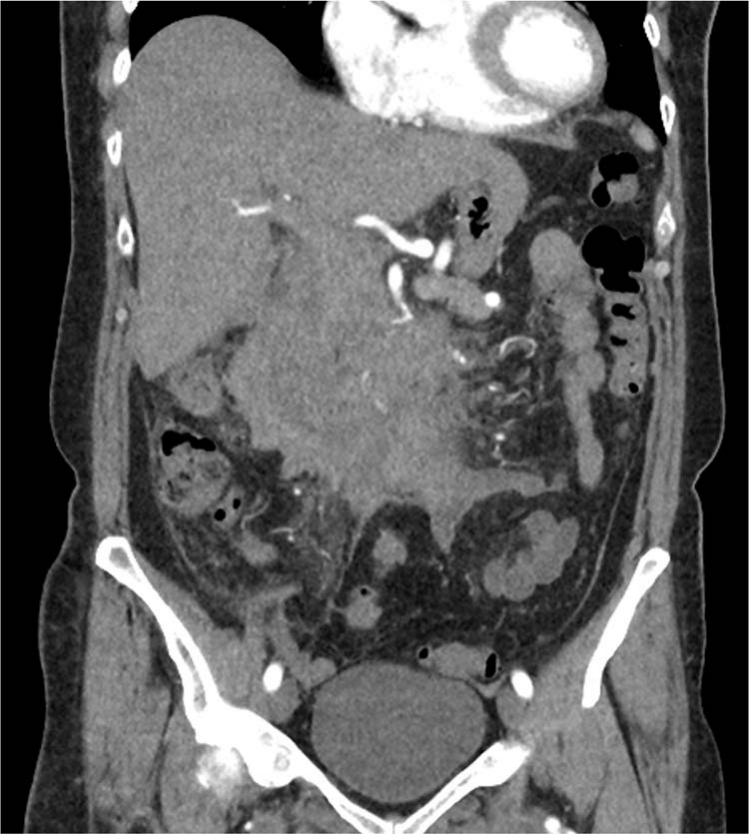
CT triple phase, coronal view. Stable periduodenal hematoma, no acute hemorrhage or vascular abnormality identified.
